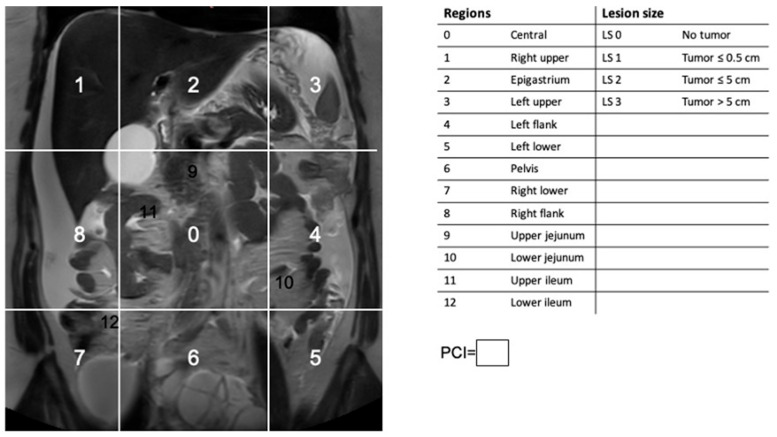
MRI-based Peritoneal Cancer Index (PCI) adapted from the laparotomic score used to determine peritoneal tumor load. The overall PCI score is obtained by adding all individual regional scores, yielding a range from 0 to 39.
